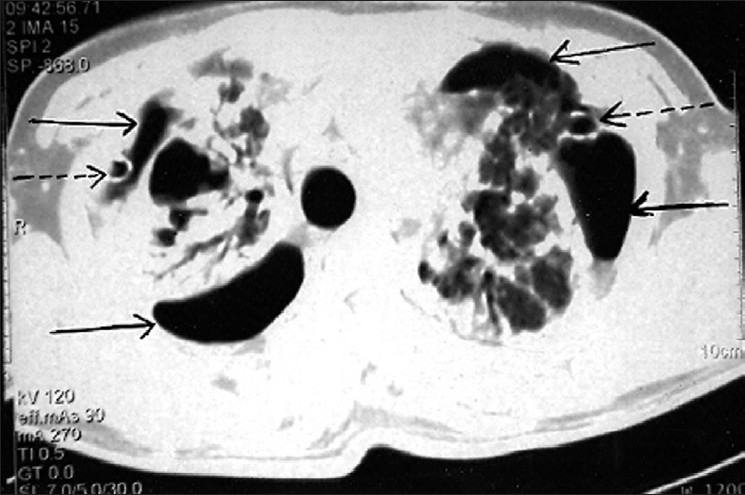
CT Thorax showing bilateral pneumothorax (solid arrows), bilateral chest tube in situ (interrupted arrows) along with reticulonodular shadows
